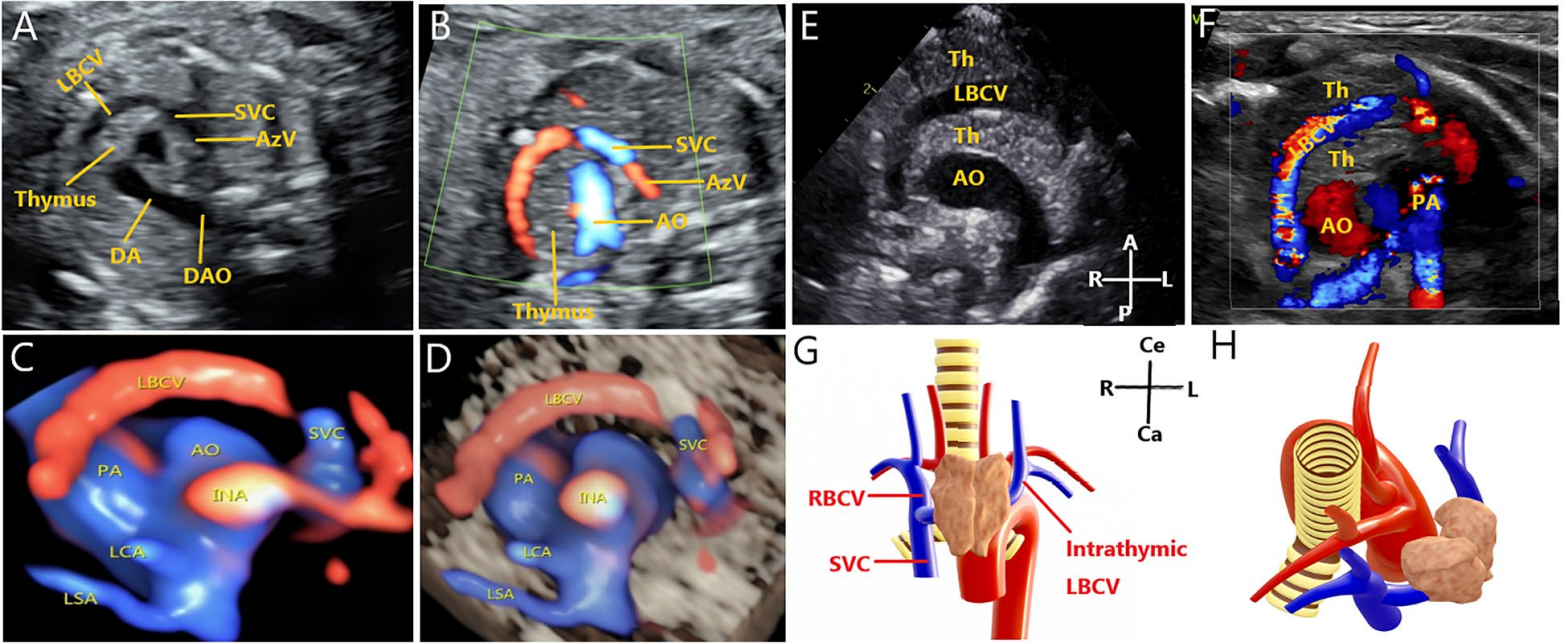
Images of the intrathymic LBCV using two-dimensional (2D) and three-dimensional ultrasonography. A 2D ultrasound image showing intrathymic LBCV. B Color Doppler blood flow demonstrating intrathymic LBCV. C-D HD-flow render mode and spatiotemporal image correlation showing that the LBCV passes through the thymus. E–F Postpartum 2D and color Doppler ultrasound images showing that the LBCV runs in the thymus, which demonstrates good consistency with the prenatal ultrasound image. G-H Structural presentation of intrathymic LBCV. G Anterior view. H Superior view. AO, aorta; PA, pulmonary artery; SVC, superior vena cava; DA, ductus arteriosus; AzV, azygos vein; DAO, descending aorta; LBCV, left brachiocephalic vein; Th, thymus; LSA, left subclavian artery; RBCV, right brachiocephalic vein; L, left; R, right; A, abdominal; P, spinal; Ce, cephalic; Ca, caudal
